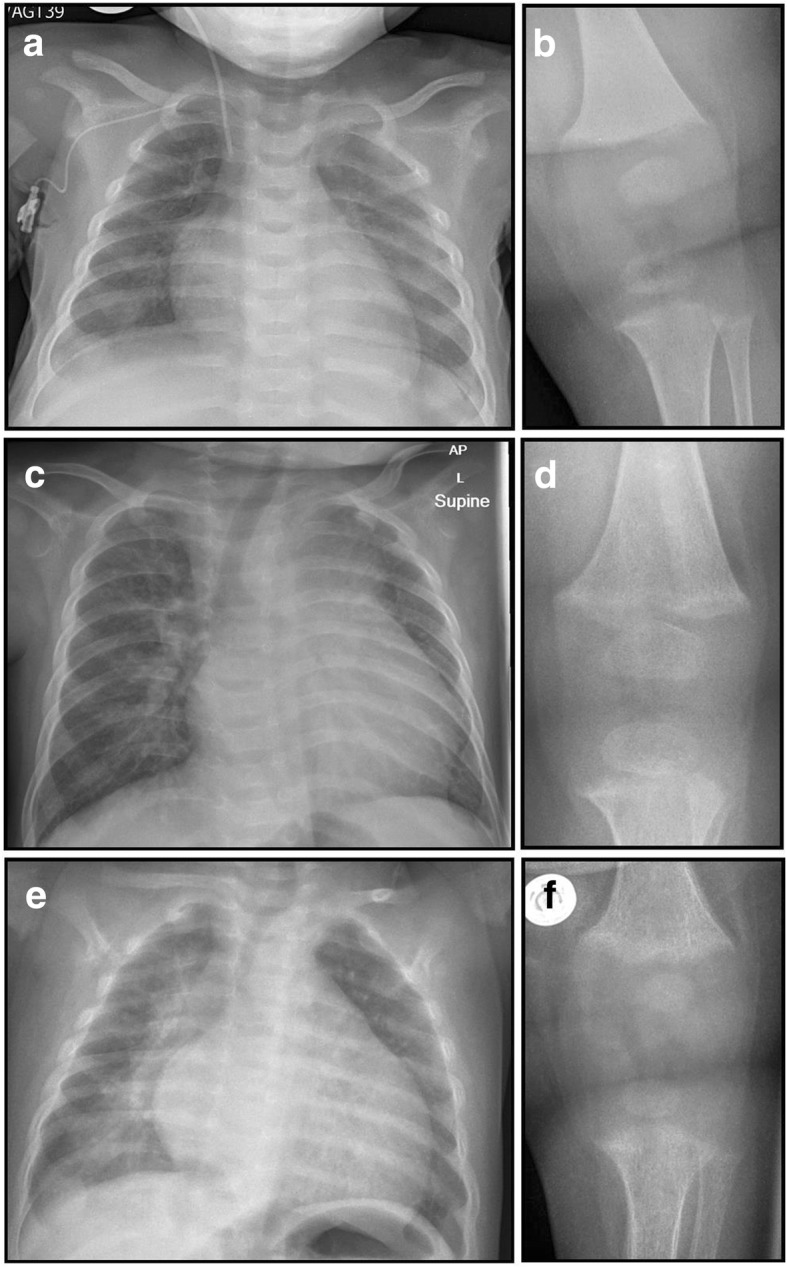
Radiographs. Chest and knee radiographs of Patient 1 (a, b), 2 (c, d) and 3 (e, f) demonstrate cardiomegaly and rickets
